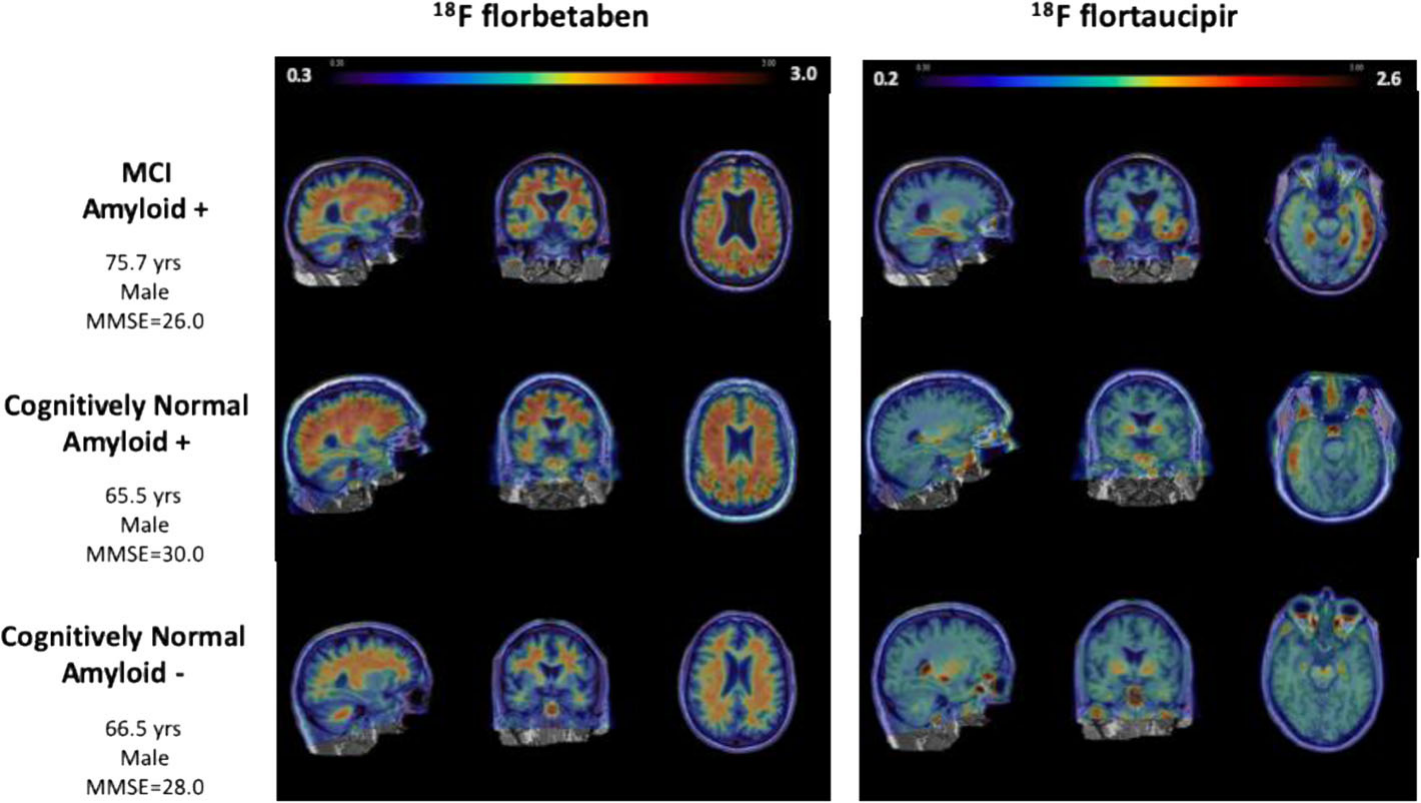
Comparison of [18F]florbetaben and [18F]flortaucipir for three patients. The authors would like to acknowledge Dr. Susan Landau (UC Berkeley) for her assistance in the creation of this figure. Scale is standardised uptake value ratio (SUVr)
